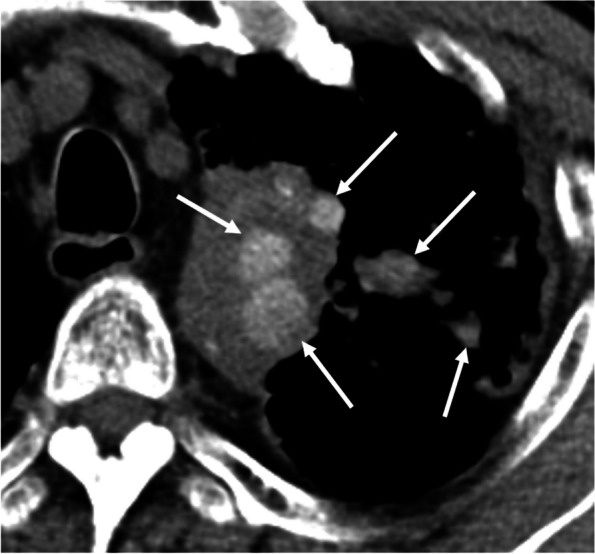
Allergic bronchopulmonary aspergillosis in a 62-year-old man. CT image shows high-attenuation mucus plugs in the left upper lobe (arrows)
